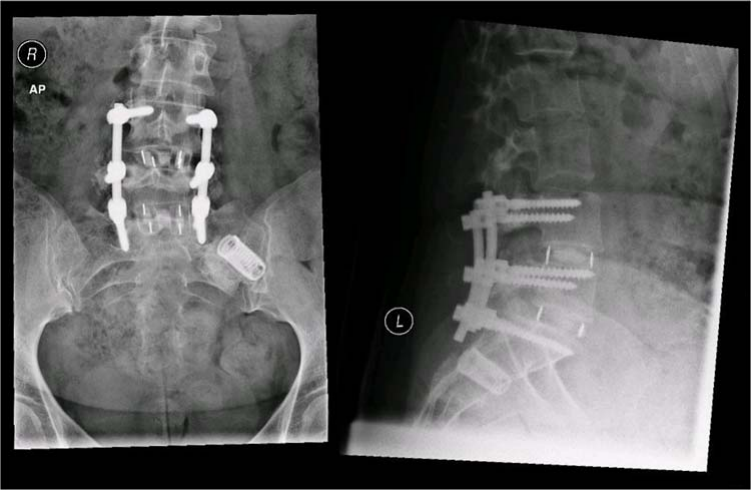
Postoperative X-ray of the pelvis following the second fusion surgery: postoperative X-ray of the pelvis, performed in January 2016 following the second fusion surgery, showing 360° (front and back) fusion of the L4/5 and L5/S1 disc space.
